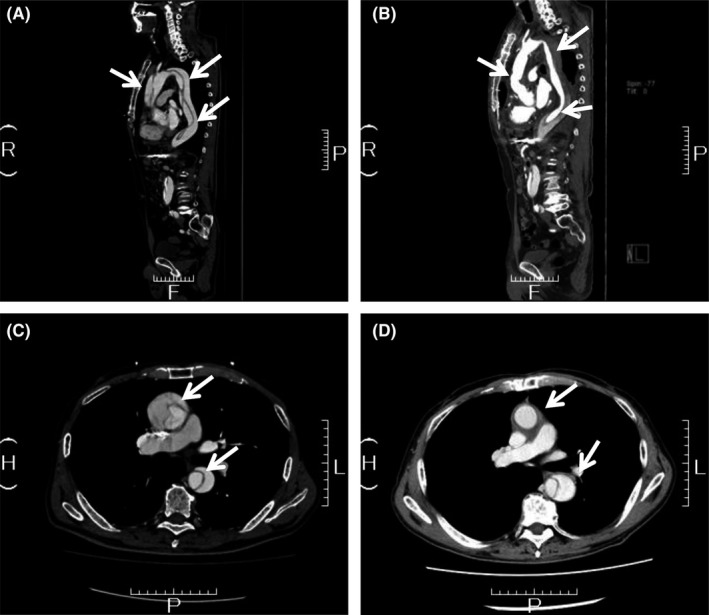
Sagittal (A and B) and transverse (C and D) views of the abdominal CT demonstrating widespread aortic dissection from the chest lesion to the abdominal lesion (A and C; before operation, B and D; 2 mo after operation)
